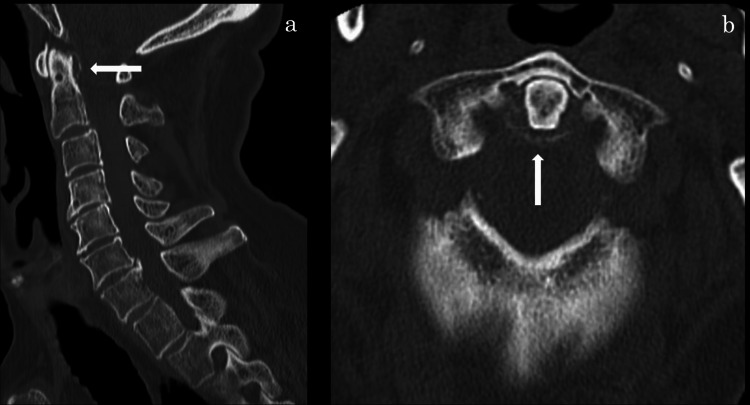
CT scan of the cervical spine
